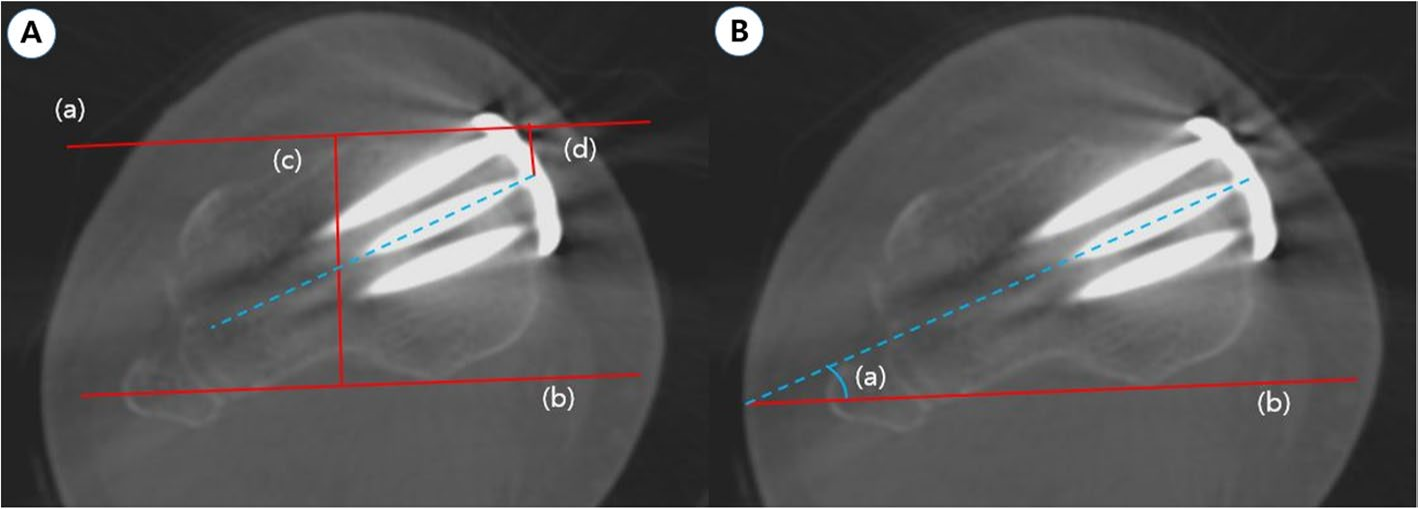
Measurement of plate position and screw angle in the proximal fragment. a A posterior line (*b*) crossing the posterior cortex of the proximal tibia was drawn and a second line (*a*) parallel to the posterior line and crossing the anterior surface of the proximal tibia was drawn. Anteroposterior (AP) position of the plate was evaluated by calculating the ratio between the distance of the anterior line to the plate center (*d*) and distance of AP proximal tibia length (*c*) was measured from the posterior line (b) to the anterior line (*a*). The plate position on the axial CT image was represented as a percentile from 0 (anterior) to 100 (posterior). b Measurement of the plate position in the screw angle. (*a*) The angle between the proximal central screw and the posterior cortex line (*b*)
